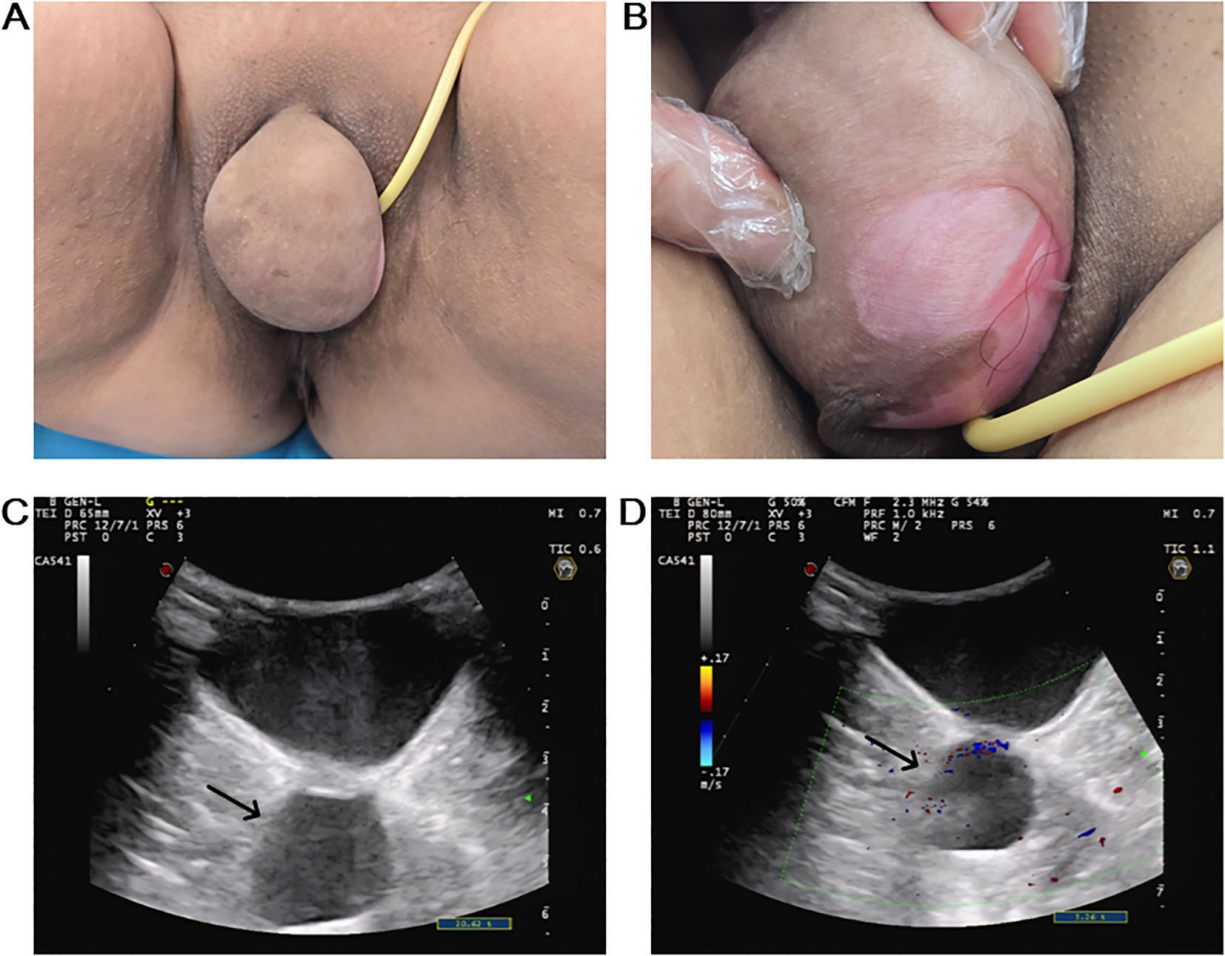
Preoperative clinical and imaging findings. (A) A giant cystic mass in the right upper labia minora obscured the urethral meatus and vaginal orifice. (B) The cyst resulted in abnormal cutaneous manifestations and lesions. (C) US showed a 8.2 × 5.8 × 3.9 cm, well defined, hypoechoic cystic mass within the right labia minora (black arrow). (D) Color Doppler flow imaging demonstrated no significant flow signal within the cyst (black arrow).
